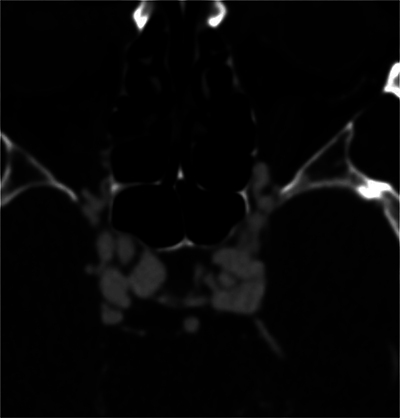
CT angiography showing early opacification of the cavernous sinuses.
